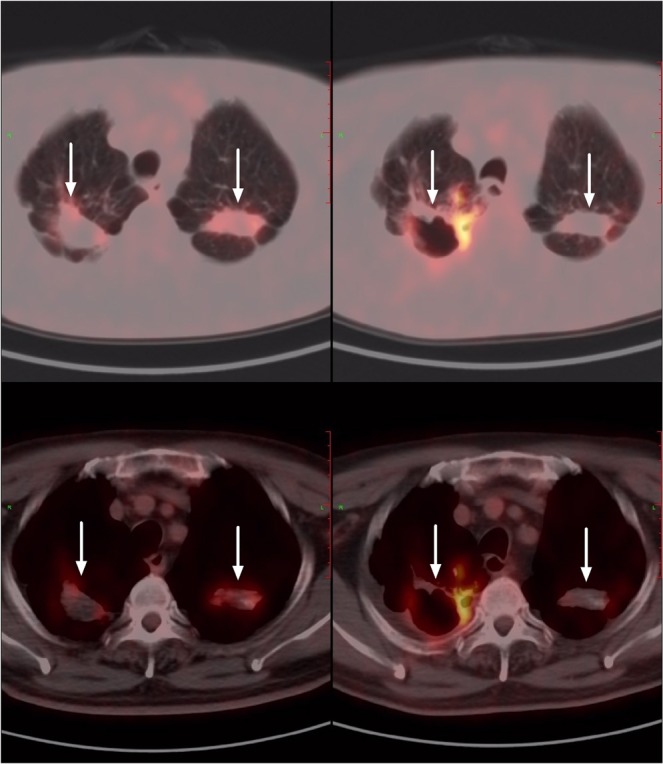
A 70-year-old man with history of colon cancer. Old tuberculosis lesions were seen at the apex of both lungs. The SUVmax = 3.1 (right) and SUVmax = 3.2 (left) on the first PET/CT scan. Six months after surgery and systemic chemotherapy, PET/CT showed that the volume of the old tuberculosis foci on the right side increased significantly. There were cavities in the lesion, new flaky dense shadows around them, and the SUVmax increased to 7.5. Interestingly, the metabolism of the left old tuberculosis decreased (SUVmax = 1.5).
